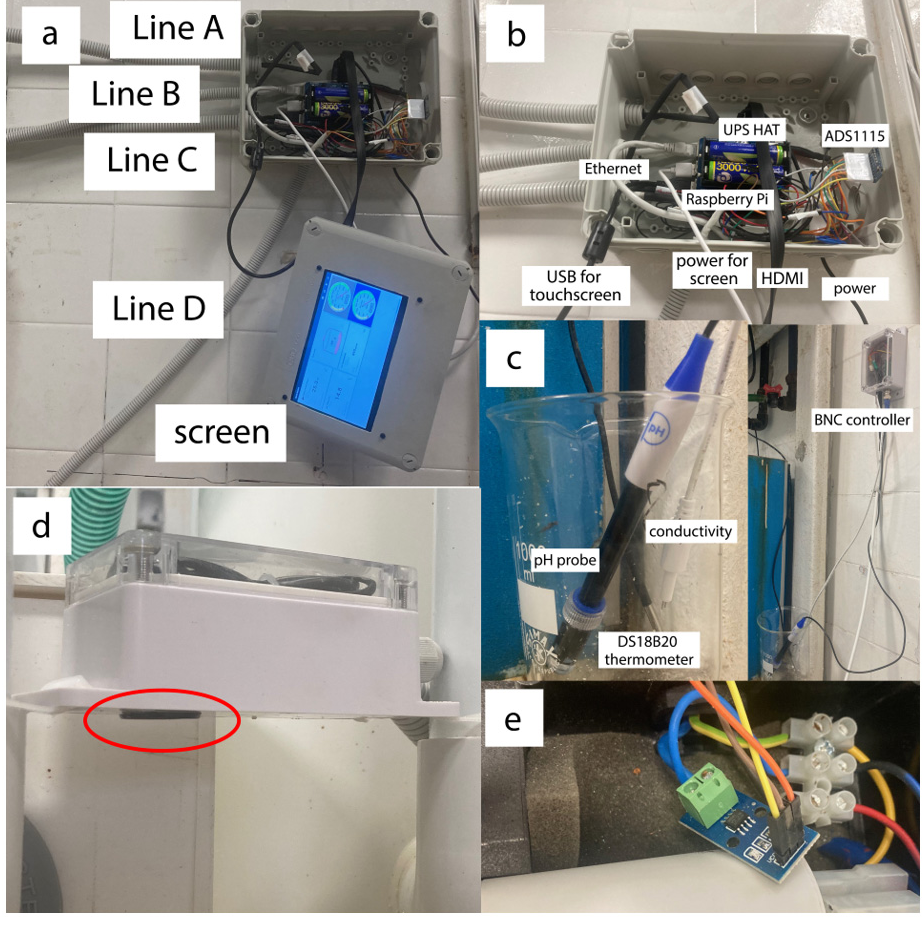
(a). The system is mounted on the wall, with a 7-inch screen. Line A connects the ultrasonic sensor, Line B connects the ACS712-20A recirculation pump sensor, Line C connects the conductivity, pH, and temperature sensors, and Line D connects the dispensing pump sensor. (b) The housing for the Raspberry Pi 3 B+ single-board computer, stacked directly on top, features a UPS HAT and an ADS1155 analog-to-digital converter for data acquisition. Network connectivity is provided via an Ethernet cable to ensure stable access. The display setup includes a dedicated USB cable for touchscreen functionality and an HDMI cable for video signal transmission. Primary power is supplied through a power supply unit connected to the UPS HAT. (c) In the left image, the thermometer, conductivity, and pH sensors are shown. In the right image, the BNC controller is depicted within its waterproof housing. (d) The ultrasonic sensor (highlighted in the red ellipse) is installed at the bottom of the waterproof housing. (e) The pump line is equipped with an ACS712-20A current sensor.
